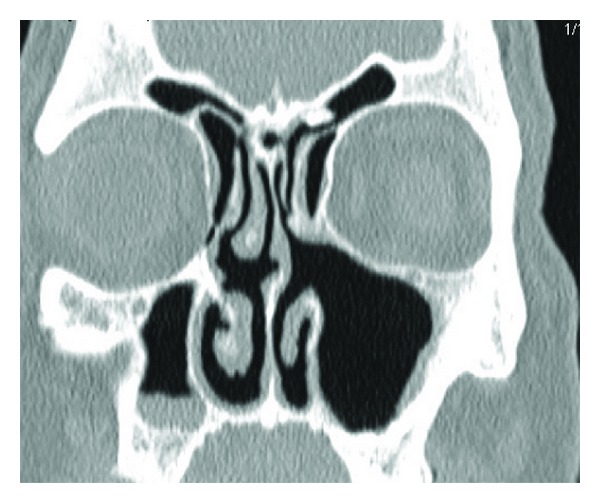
A coronal CT scan after ESS, showing remodeling of the orbital floor.
